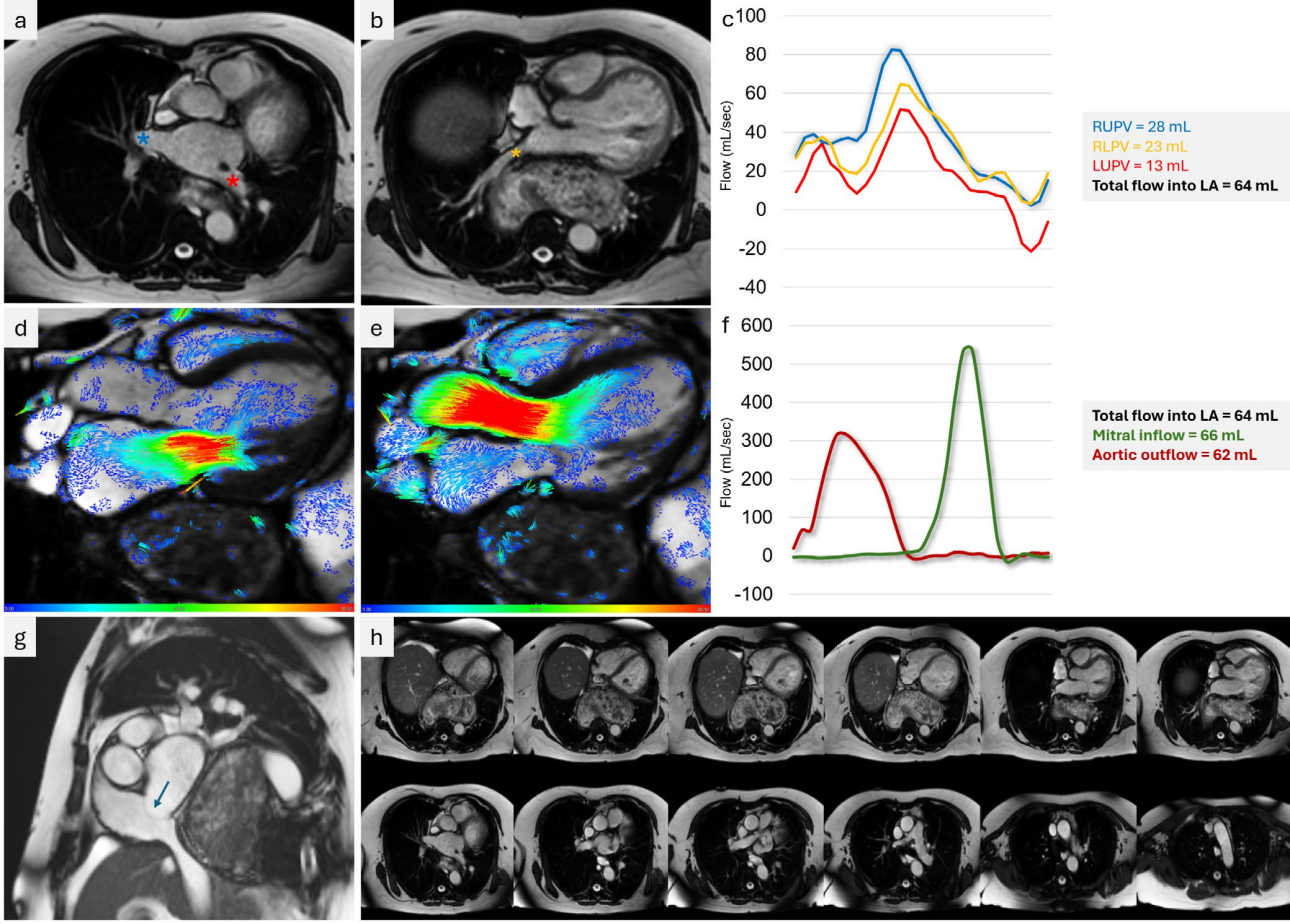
4D Flow CMR and anatomical evaluation of pulmonary veins, mitral/aortic dynamics and hiatal hernia‐mediated left atrial compression. (a) Axial T1‐weighted cardiac MRI at upper pulmonary vein (PV) level showing right upper PV (blue asterisk) and left upper PV draining into the left atrium (LA). (b) Same plane at lower PV level with right lower PV (yellow asterisk) adjacent to the hiatal hernia (HH). (c) Time‐resolved flow curves through RUPV, RLPV and LUPV: D > S pattern (dominant diastolic over systolic flow) consistent with elevated LA pressure. Volumetric return into LA totals 64 mL per cycle. (d–e) 4D‐flow streamline visualizations overlaid on cine imaging showing mitral inflow (d) and aortic outflow (e) over the same timeframe. (f) Flow‐time curves from panels (d–e): Mitral inflow 66 mL, aortic outflow 62 mL (balanced with LA input). (g) Sagittal MRI short‐axis view demonstrating external LA compression by HH (arrow). (h) Axial thoracic stack showing large HH with mediastinal mass effect, impinging on PV and LA anatomy. 4D‐flow MR, four‐dimensional flow magnetic resonance imaging; HH, hiatal hernia; LA, left atrium; LUPV, left upper pulmonary vein; PV, pulmonary vein; RUPV, right upper pulmonary vein; RLPV, right lower pulmonary vein.
